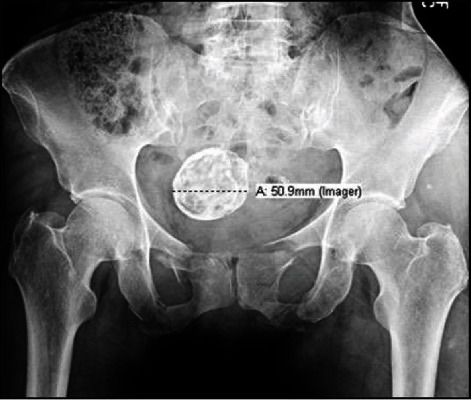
Initial anteroposterior pelvis X-ray showing bilateral pubic rami fractures and a large uterine fibroid, in a 65-year-old patient pedestrian struck by a motor vehicle.
